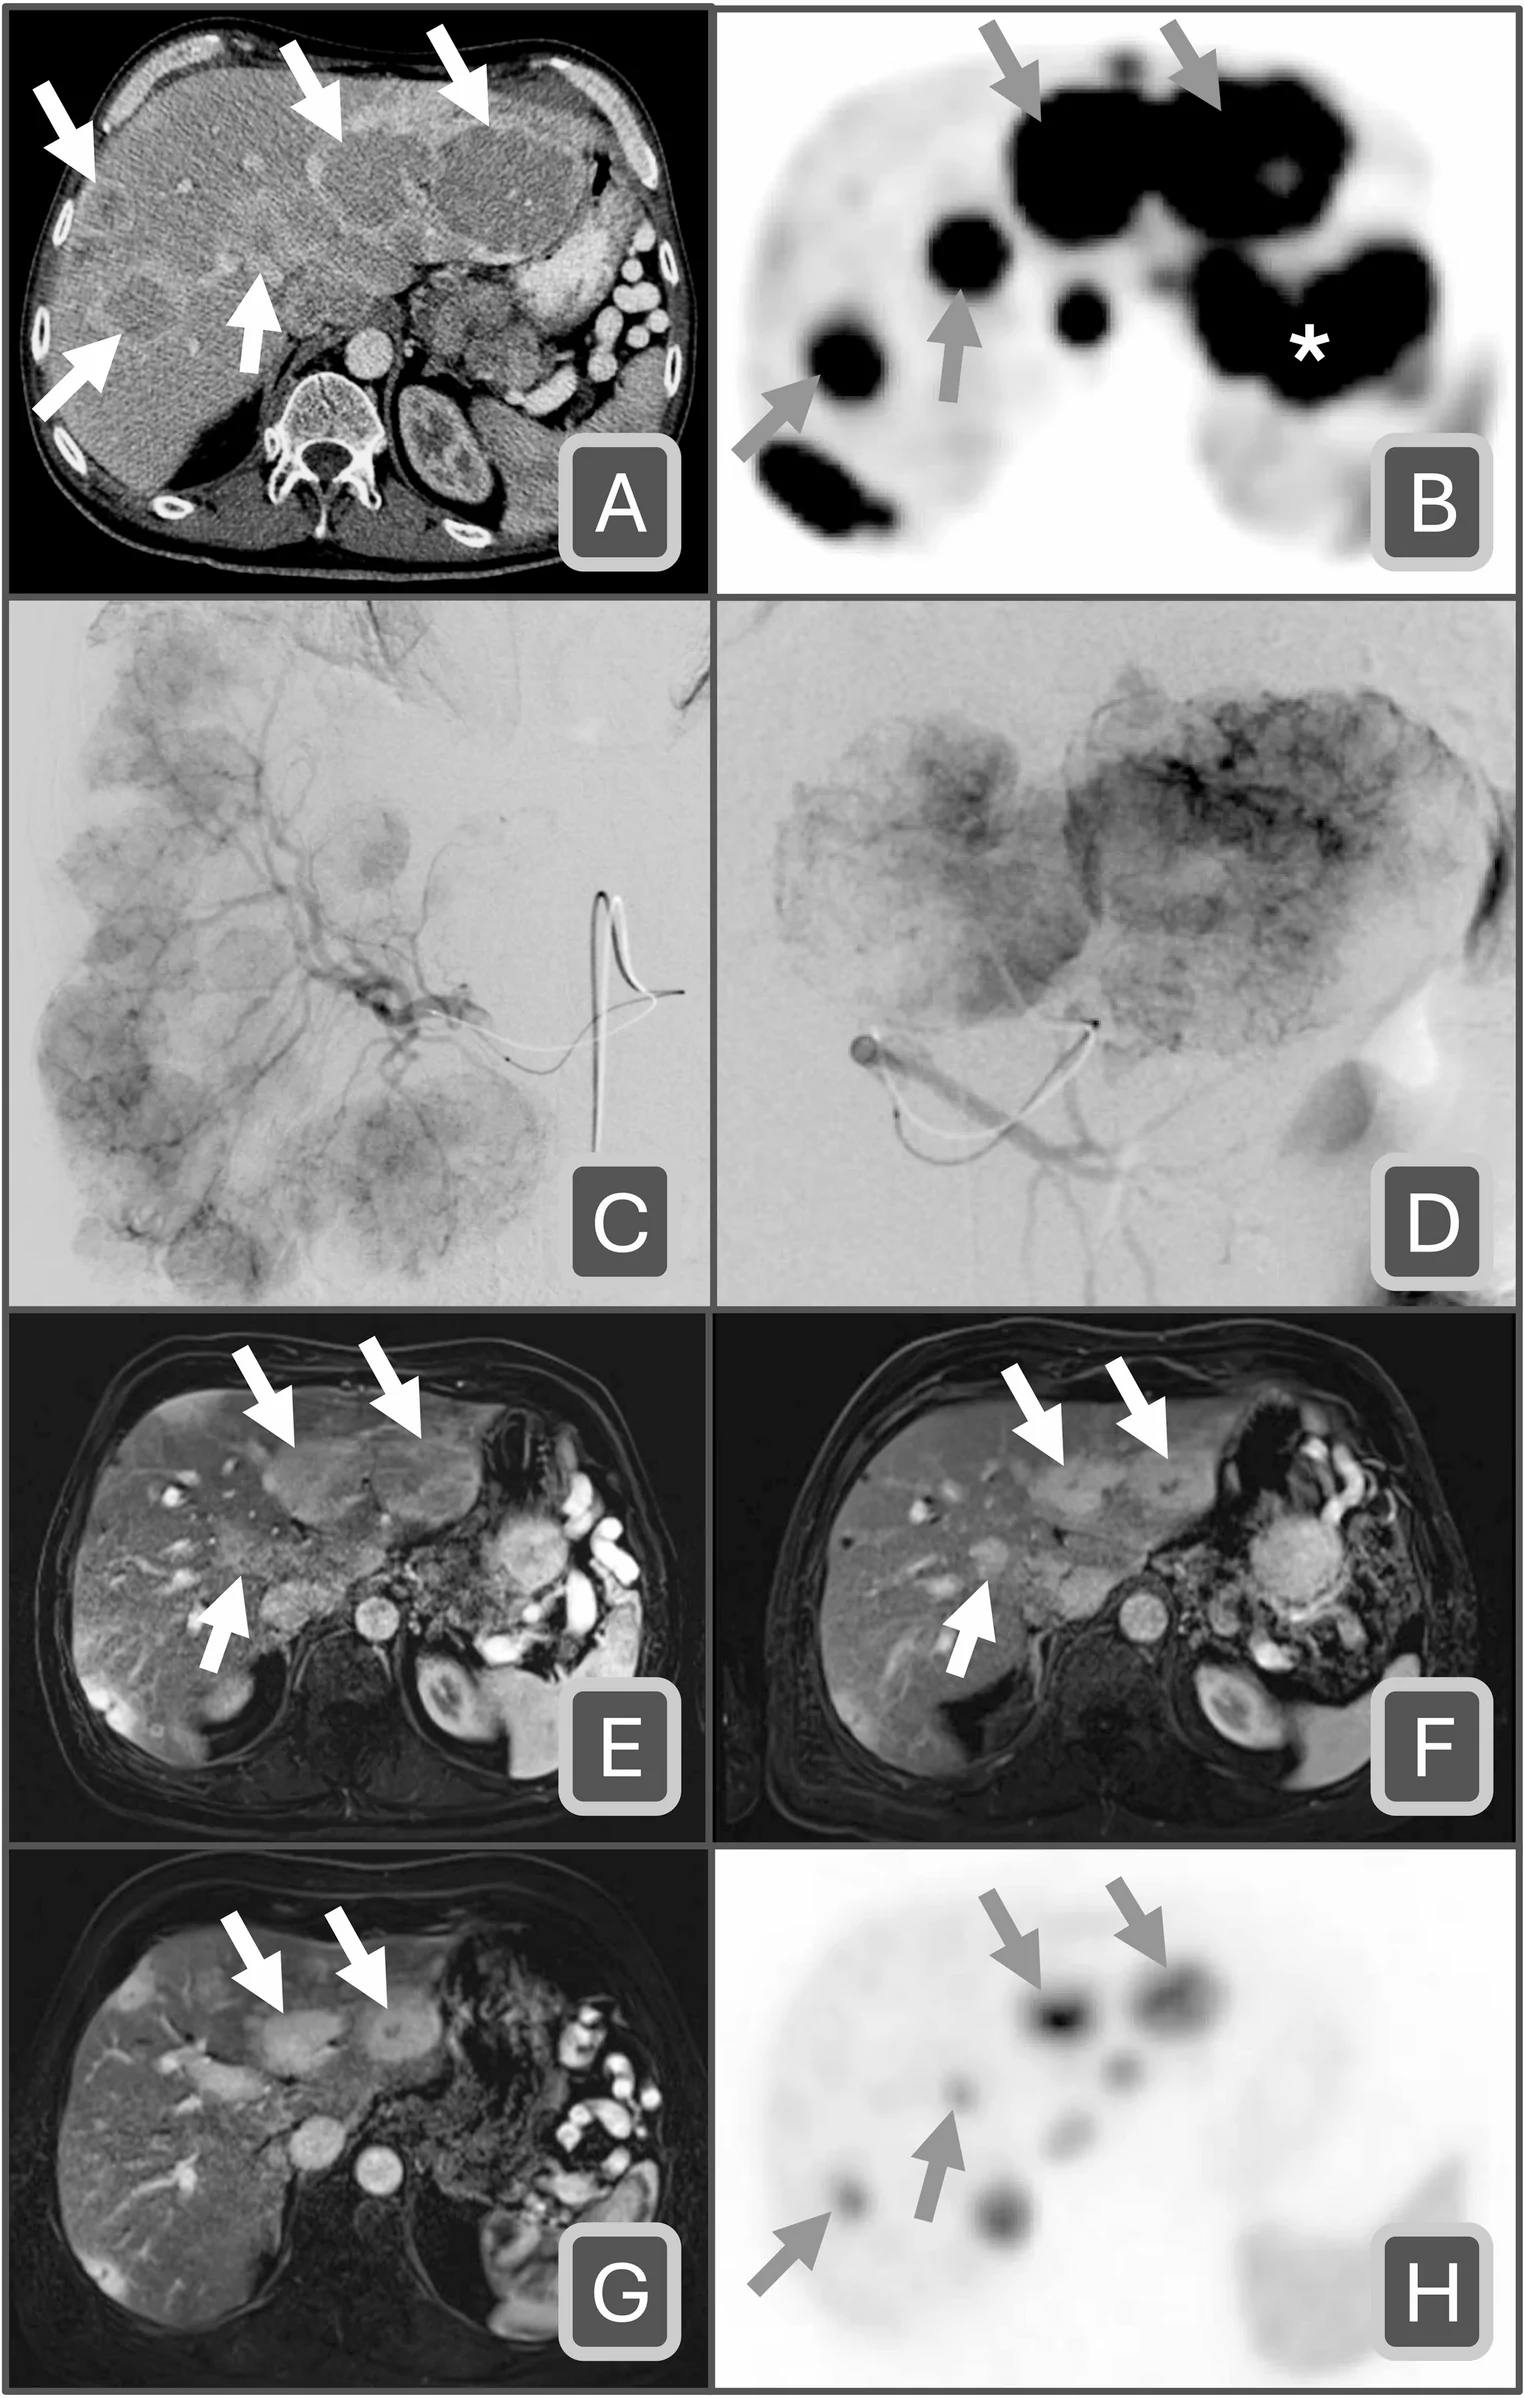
54 year-old with grade 2 metastatic pancreatic neuroendocrine tumor treated with the combination CAPTEM-y90 regimen. CAPTEM was administered for 12 cycles over 12 months, with right hepatic TARE performed with the second cycle and left hepatic TARE with the third cycle. (A) Pre-treatment arterial-phase CT demonstrates bulky bilobar liver metastases (white arrows). (B) Pre-treatment DOTATATE PET-CT redemonstrates the patient’s bulky bilobar liver metastases (gray arrows). The primary pancreatic tumor is also seen (white asterisk). (C) Right hepatic arteriogram performed during pre-y90 mapping demonstrates hypervascular right hepatic neuroendocrine tumor metastases. (D) Left hepatic arteriogram performed during pre-y90 mapping demonstrates hypervascular right hepatic neuroendocrine tumor metastases, corresponding to the bulky left side lesions seen on pre-procedural imaging. (E) 3-month follow-up subtracted early arterial phase MRI demonstrates a partial response in the treated NETLMs (white arrows) by both RECIST and mRECIST criteria. (F) 12-month follow up subtracted early arterial phase MRI demonstrates further decrease in size of the treated NETLMs (white arrows), with continued partial response. CAPTEM was stopped at this time. (G) 21-month follow up subtracted early arterial phase MRI demonstrates stability of treated NETLMs (white arrows). (H) 24-month follow-up DOTATATE PET-CT demonstrates significant shrinkage of NETLMs (gray arrows) compared to pre-treatment PET-CT (as demonstrated in figure part B), consistent with a sustained partial response throughout the 24 month follow-up period
